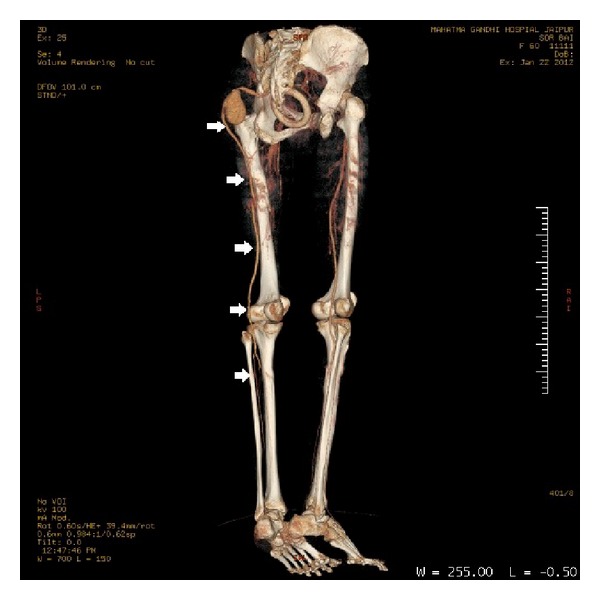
Volume rendering image of CT angiogram. The arrows show the course of persistent sciatic artery through the thigh and its distal run-off to the popliteal artery. Note that the popliteal artery of the opposite limb is supplied by the superficial femoral artery.
